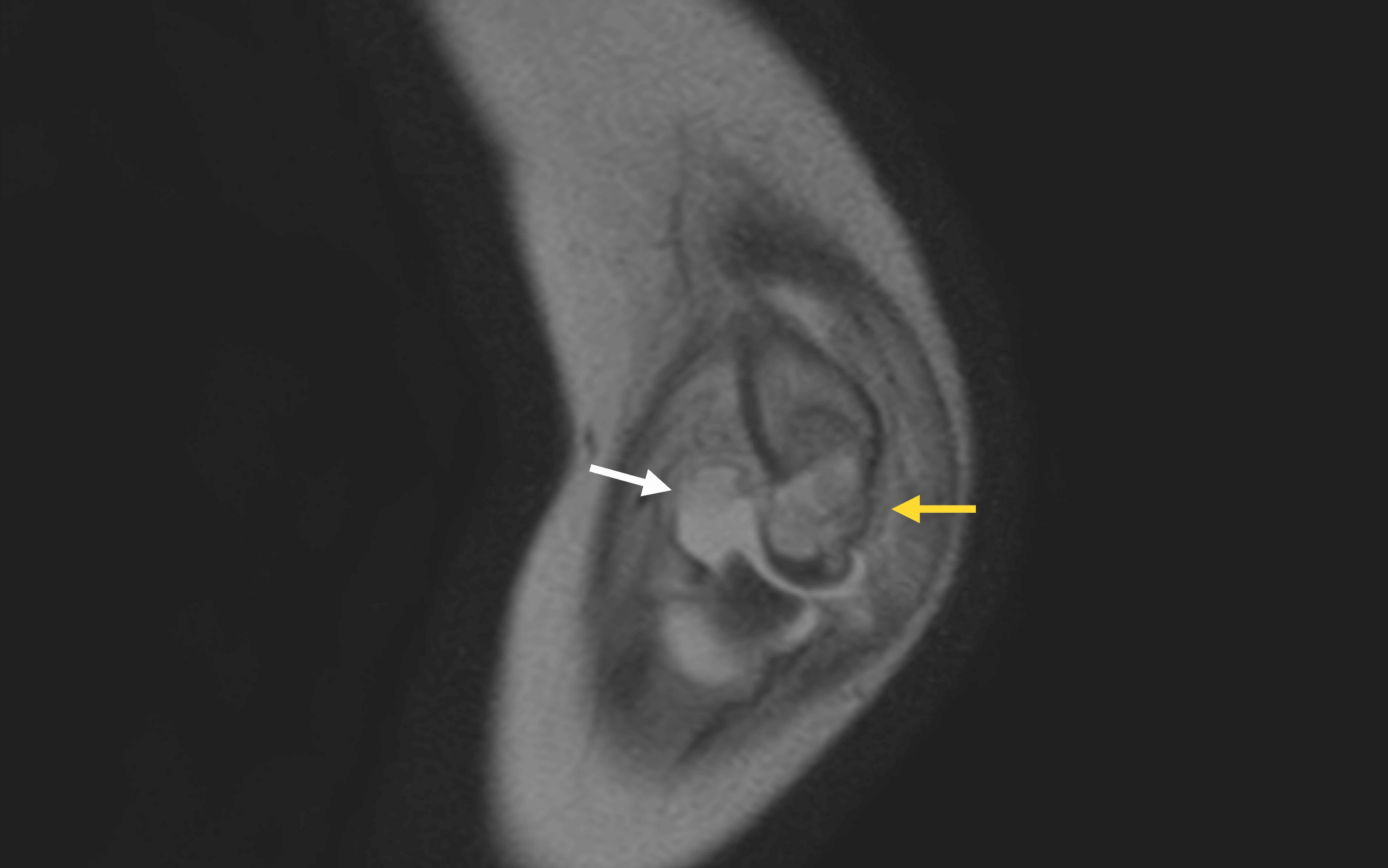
Coronal MRI indicating osteomyelitis of the distal humerus Coronal MRI of the right elbow showing joint effusion (white arrow) and marrow hyperintensity within the distal humerus (yellow arrow), findings consistent with osteomyelitis.
